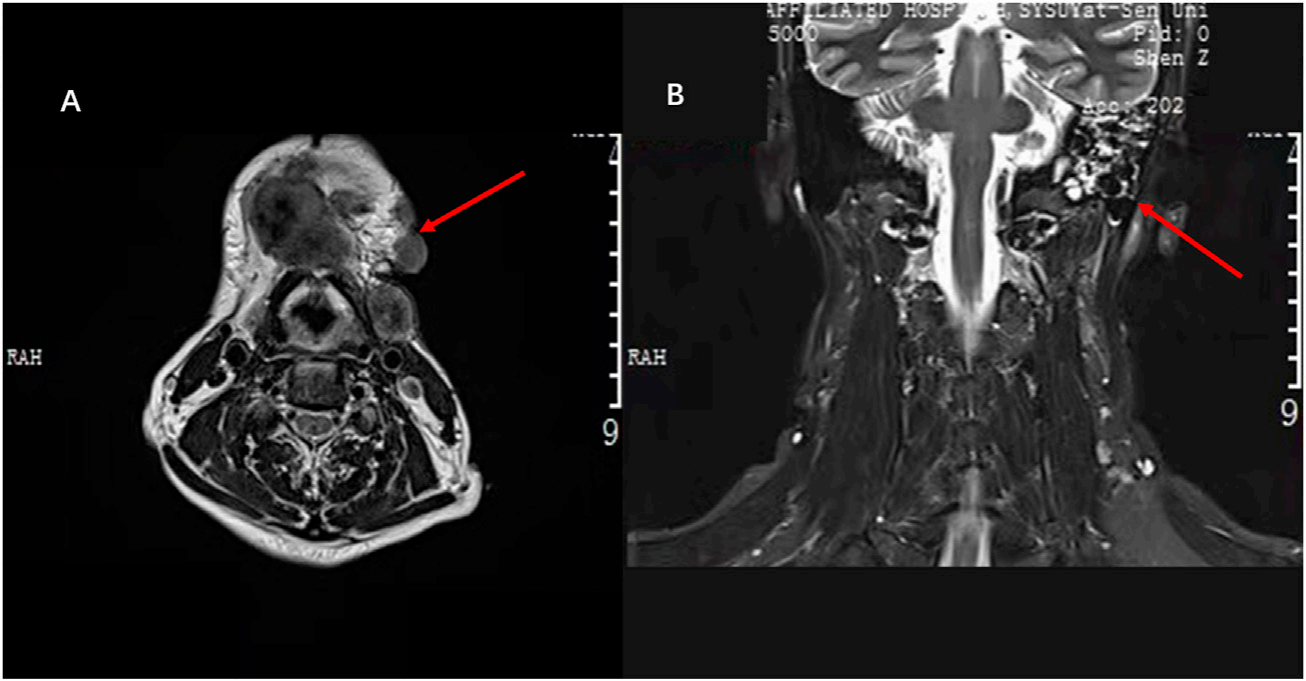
MRI image of metastatic melanoma. (A) T2-weighted axial image shows soft tissue mass with ill-defined borders involving the left lateral wall and cervical lymph node metastasis. The lesion is causing compression of oropharynx. (B) Post contrast T2-weighted coronal image shows the enlarged cervical lymph node.
